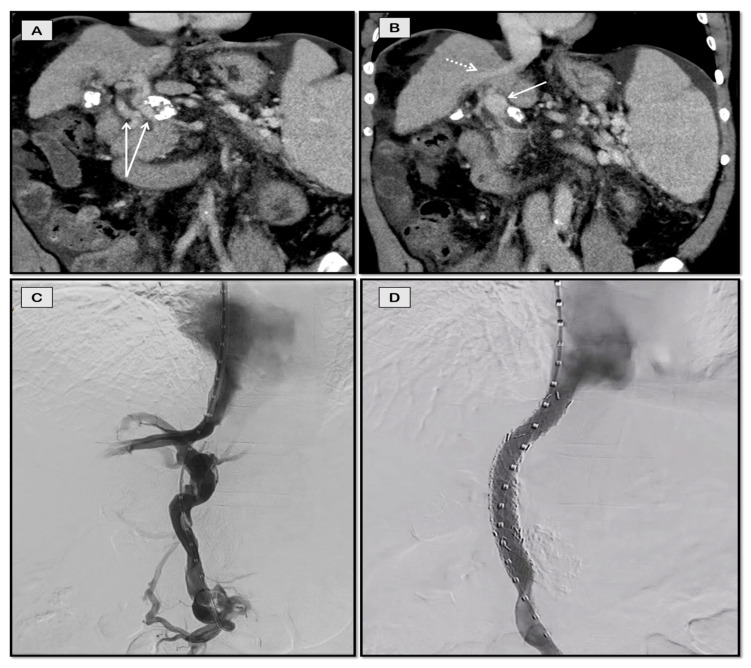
TIPS through collateral vein for refractory ascites in a 59-year-old man with chronic portal vein thrombosis secondary to NASH-cirrhosis. Coronal-oblique CT images (A,B) showing portal cavernoma (arrows in A) and dominant collateral vein (solid arrow in B) in the same plane as middle hepatic vein (dashed arrow in B). Collateral vein was accessed through jugular route (C) and stented with restoration of flow (D).
